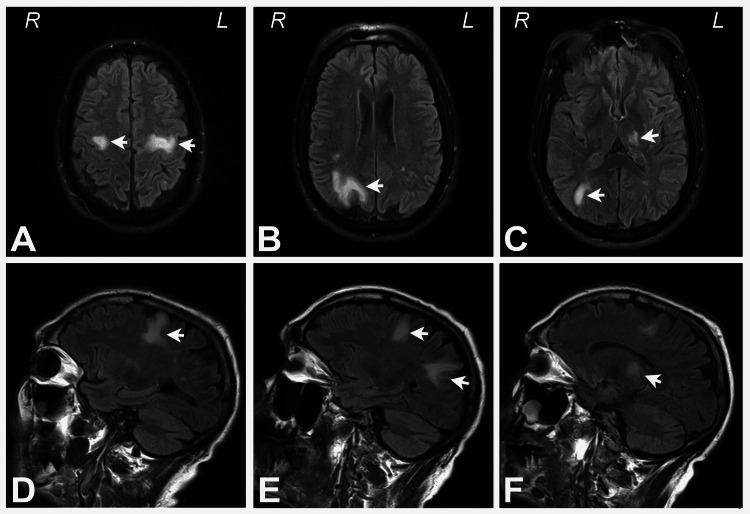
Unenhanced FLAIR axial and sagittal images demonstrating bifrontal, right parietal, and bilateral thalamic lesions Top row: Unenhanced FLAIR axial images demonstrating the (A) bifrontal, (B) right parietal, and (C) left thalamic and right thalamic lesions (arrows). Bottom row: Unenhanced FLAIR sagittal images showing the (D) left posterior frontal, (E) right posterior frontal and right parietal, and (F) left thalamic lesions (arrows).
